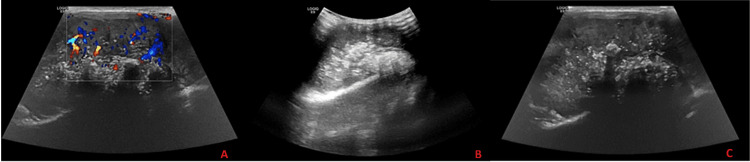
Ultrasonography images of the left scapula showing a heterogenous mass in the back of the left shoulder measuring 9.7 cm x 5 cm with (A) internal vascularity, (B) scattered calcifications, and (C) calcification
